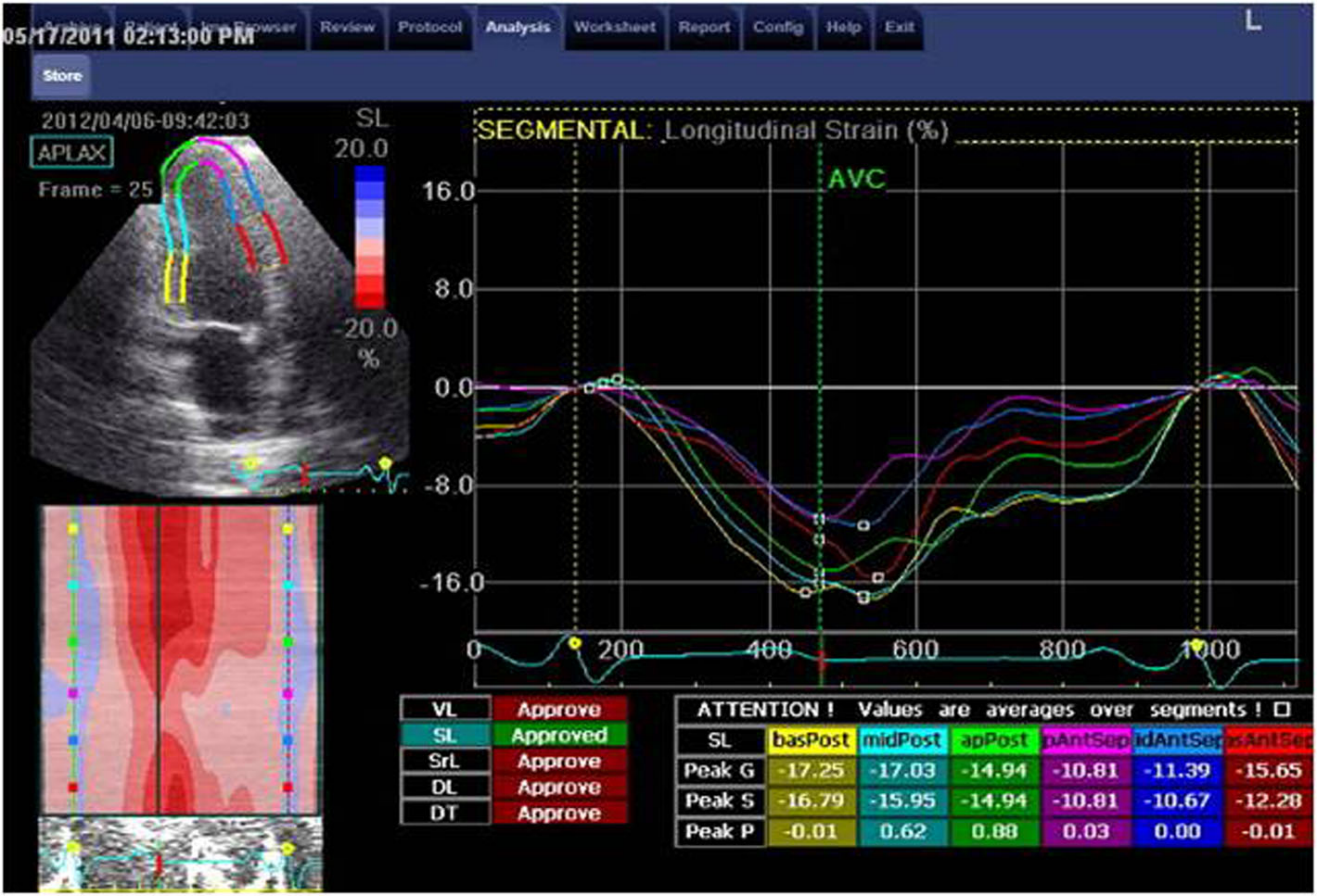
Apical three-chamber view and analysis of posterior and anteroseptal wall strain using TTE
